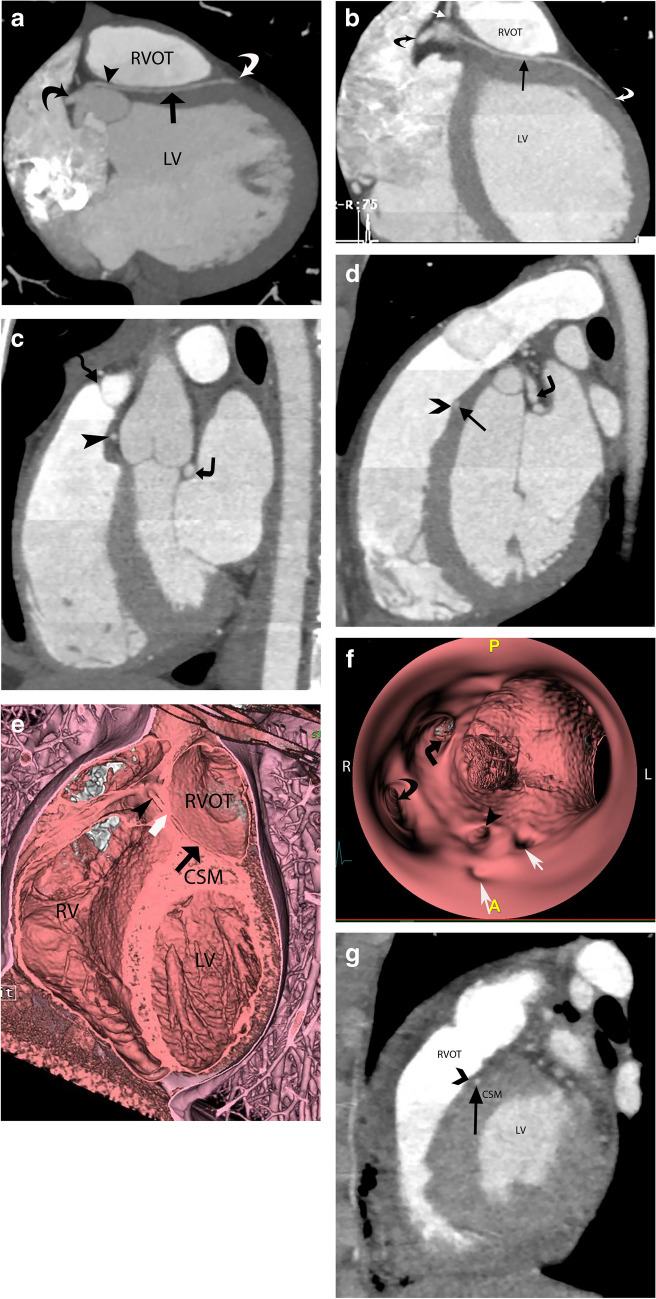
A 17-year-old male with a cardiac murmur and anomalous aortic origin of the left anterior descending artery (LAD) from the right sinus with a transseptal course. a Coronary CT angiography (CTA), oblique axial image. LAD has an anomalous origin from the right sinus of Valsalva, after which it takes a sharp turn towards the left, coursing between the aorta and right ventricular outflow tract (RVOT). A black arrowhead points to the extraconal segment of LAD. The LAD extends into the conal septal myocardium (black arrow), defining its transconal course, and then exits out at the epicardial surface (white curved arrow). The right coronary artery (RCA) (curved black arrow) has a normal origin. LV, left ventricle. b Coronary CTA, oblique coronal image. After its anomalous origin, the LAD extends into the conal septal myocardium, defining its transconal segment (black arrow) until it extends to the epicardial surface (curved white arrow). The RCA (curved black arrow) and conus artery (white arrow) originate from the right sinus of Valsalva. LV, left ventricle. c Coronary CTA, oblique sagittal image. The anomalously originating LAD (black arrowhead) courses below the level of the pulmonary annulus (wavy black arrow) between the aorta and RVOT in the extraconal space, maintaining its round luminal caliber. There is a benign retroaortic course of the LCX (right-angled black arrow). d Coronary CTA, oblique sagittal image in systolic phase (35% of RR interval). The LAD (black arrow) courses through the conal septal myocardium, with at least 1 mm overlying septal myocardium (black chevron). Due to myocardial compression, this segment’s luminal caliber is no longer round. The retroaortic course of the LCX is also noted (right-angled black arrow). There is a tangle of vessels associated with LCX due to coronary artery fistula with the pulmonary artery in this patient (not discussed here). e Coronary CTA, endoluminal view after virtual removal of the anterior aspect of the heart and anterior wall of RVOT. The black arrowhead shows an extraconal course of the anomalously originating LAD from the opposite sinus. The block white arrow points to the entry point of the LAD into the conal septal myocardium. The LAD follows a transconal course shown by the black arrows through the conal septal myocardium (CSM). RV, right ventricle. f Coronary CTA, endoluminal view of the aortic root obtained with the camera placed in the right sinus of Valsalva pointing towards the ostium of the anomalously originating LAD from the right sinus of Valsalva. The black arrowhead points to the ostium of LAD, originating within the right sinus of Valsalva. It has a round caliber with end-on visualization of its lumen without a slitlike ostium. Five ostia are located within the right sinus of Valsalva, including the LCx (right-angled black arrow), RCA (curved black arrow), LAD (black arrowhead), and two conus arteries (white arrows), in the order of right posterior to right anterior location. A, anterior; P,  posterior; R, right; L, left. g Coronary CTA after transconal unroofing of the LAD. Oblique sagittal image in systolic phase (30% of RR interval). The anomalously originating LAD (black arrow) courses posterior to the right ventricular outflow tract (RVOT). The LAD is anterior to the conal septal myocardium (CSM), covered anteriorly by the pericardial patch (black chevron) after transconal unroofing, and hence maintains a round luminal shape in the systolic phase compared to the preoperative imaging (Fig. 3d). LV, left ventricle
